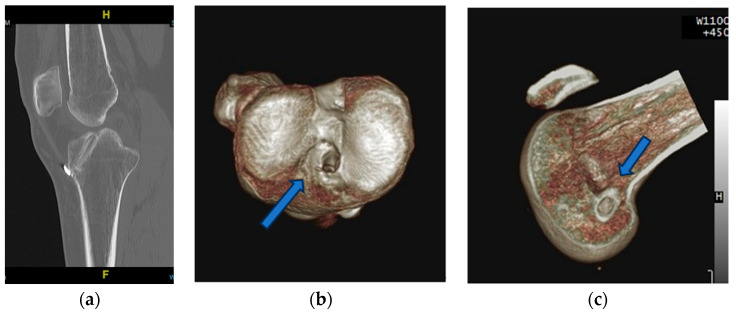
CT of right knee joint: (a) No tunnel widening was noted. (b,c) Previous tunnel positions were ideal. (the arrow represents the original bone tunnel).
